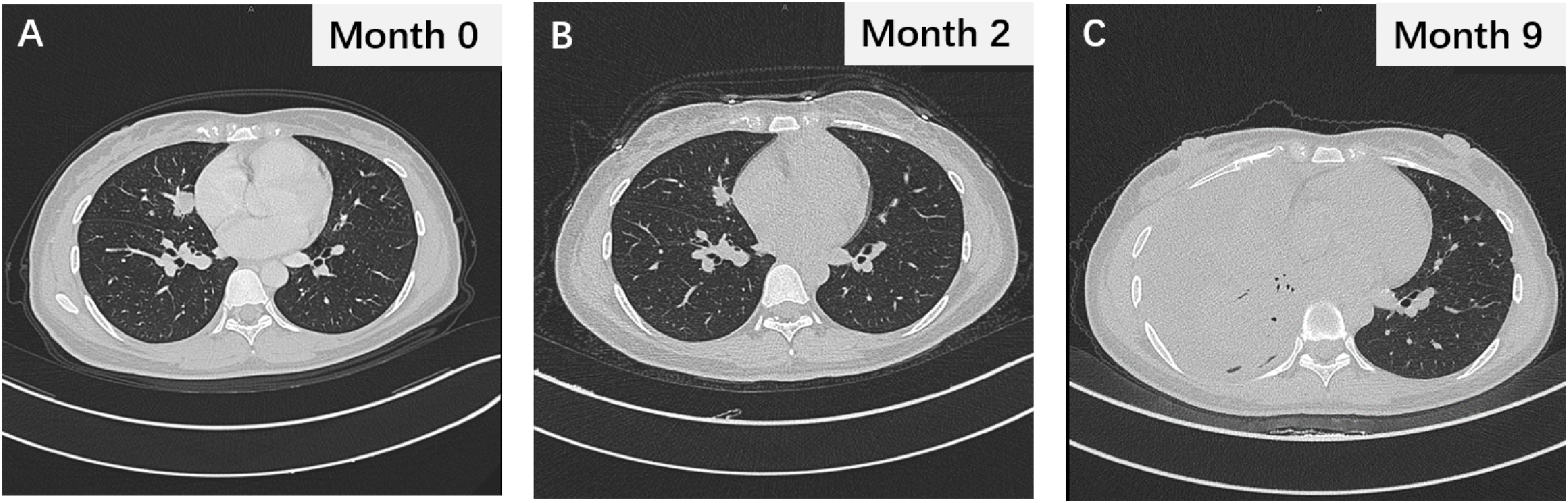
(A) The thoracic CT image displaying lung lesions during initial evaluation. (B) The thoracic CT scan showing the changes in lung lesions after 2 months of gefitinib treatment. (C) The chest CT image during gefitinib resistance.
